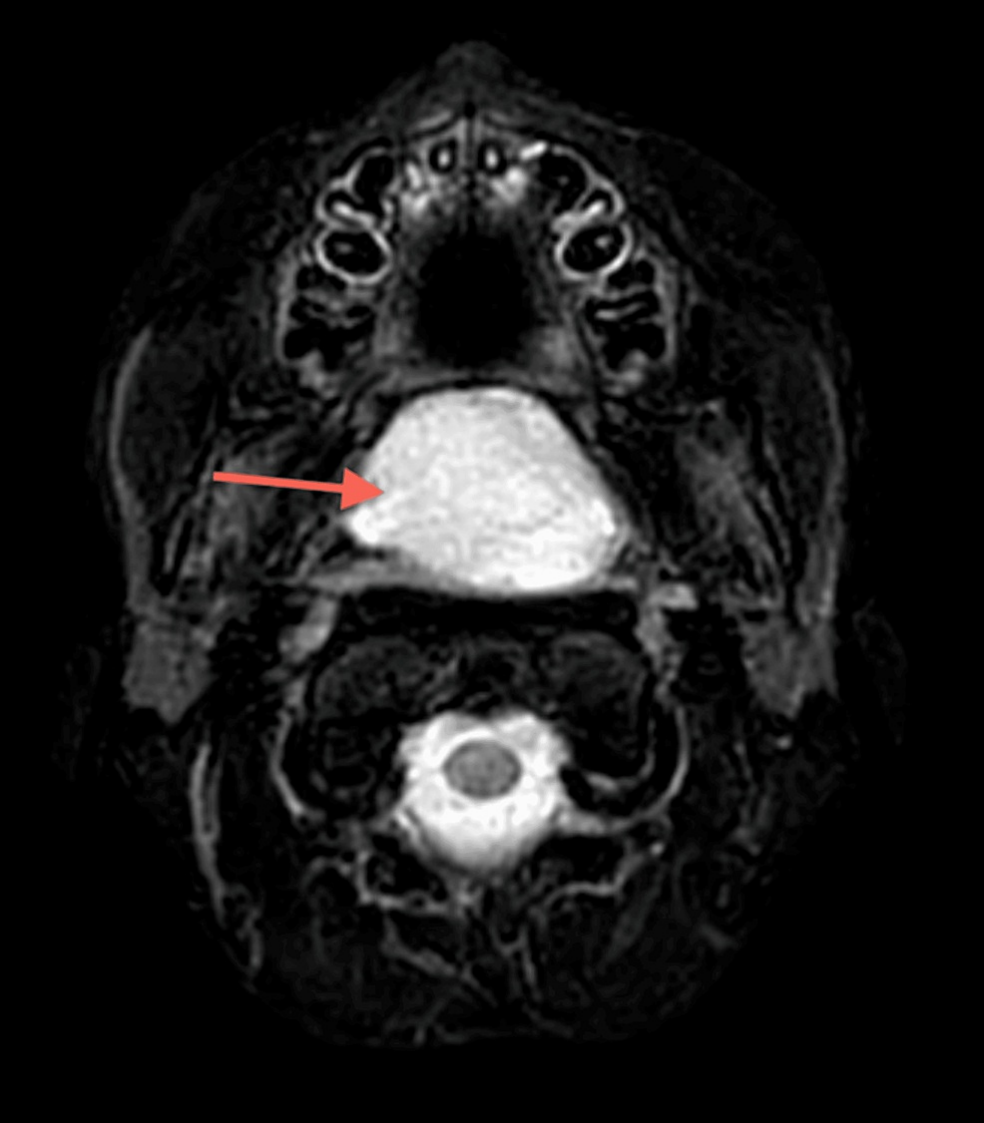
MRI Neck (T2) The large nasopharyngeal well-circumscribed cyst measures 47 x 28 x 35 mm. The sheer volume of this Thornwaldt cyst is remodeling the undersurface of the sphenoid sinus. The cyst is anteriorly eroding the posterior edge of the hard palate and midline vomer segment of the nasal septum, the uvula is displaced significantly into the oral cavity. Parapharyngeal recesses are preserved. No intracranial extension.
